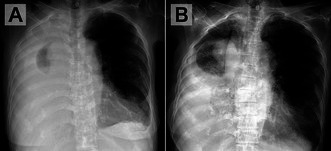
An 84‐year‐old woman was admitted for evaluation of two months of general fatigue and a right‐sided pleural effusion (PE) (Figure 1A). On admission, mild hypoxemia with an oxygen saturation of 93% on ambient air was observed. During thoracentesis, no air aspiration or changes in vital signs was observed; however, the patient developed right anterior chest pain, prompting discontinuation of the procedure after 900 mL drainage. A post‐thoracentesis chest radiograph demonstrated a hydropneumothorax (Figure 1B).
